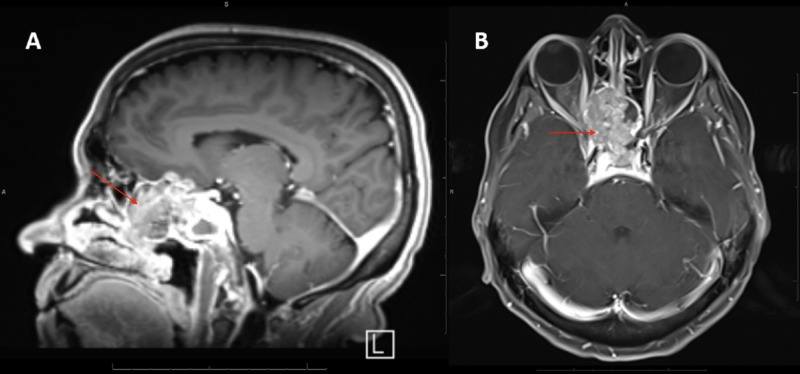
T1-enhanced magnetic resonance imaging (MRI) with fat suppression showing sinonasal squamous cell carcinoma (SCC) of the ethmoid air cells extending into the adjacent ethmoid sinus A: Sagittal view; B: Axial view.
